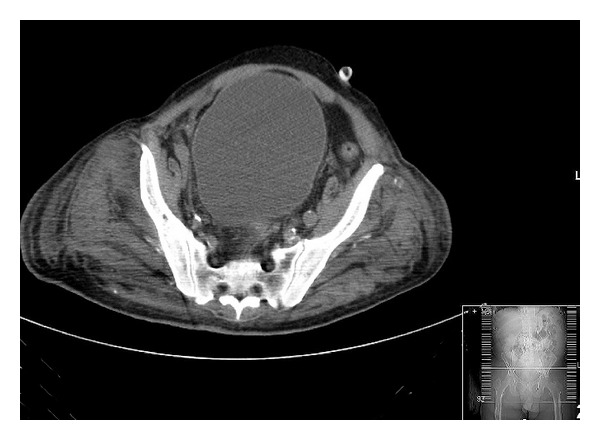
Patient 2: CT showing a grossly distended bladder blocking detailed visualization of the urethral catheter's location.
